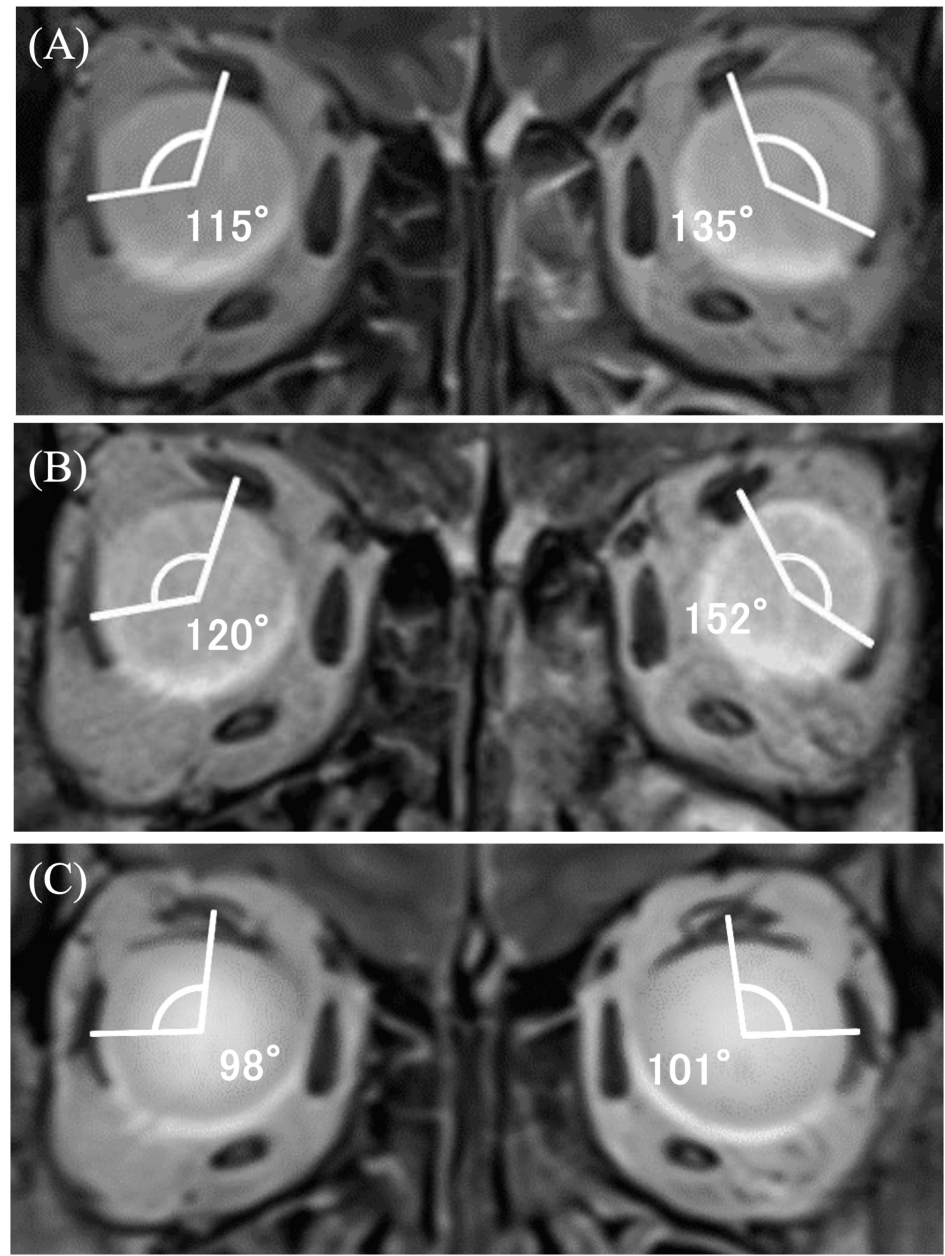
Coronal section MRI images at initial visit, before operation (seven years after initial visit), and after operation SR: superior rectus muscle; LR: lateral rectus muscle (A) is the image at the time of the initial examination, (B) is the image before surgery (seven years after the initial examination), and (C) is the image after surgery. Nasal displacement of the SR and downward displacement of the LR were observed in both eyes from the initial examination, with the angle of dislocation between the SR and the LR being 115° in the right eye and 135° in the left eye. The preoperative angle of dislocation was 120° in the right eye and 152° in the left eye, but five months after surgery, the angle of dislocation of the right and left eyes was 98° and 101°, respectively, and the angle of dislocation also showed improvement
